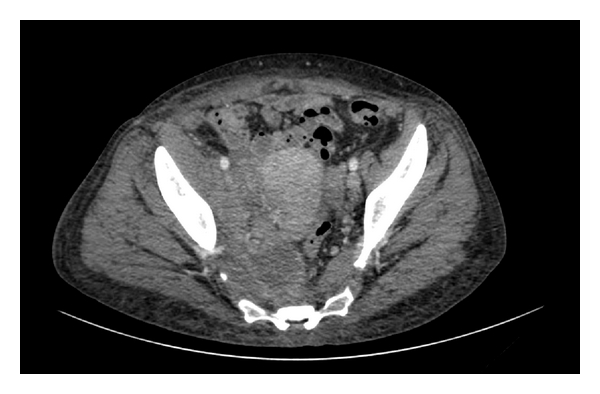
Computed tomography (CT) scan showed the mass occupying the extraperitoneal space infiltrating the psoas muscle posteriorly and the pelvis anteriorly.
